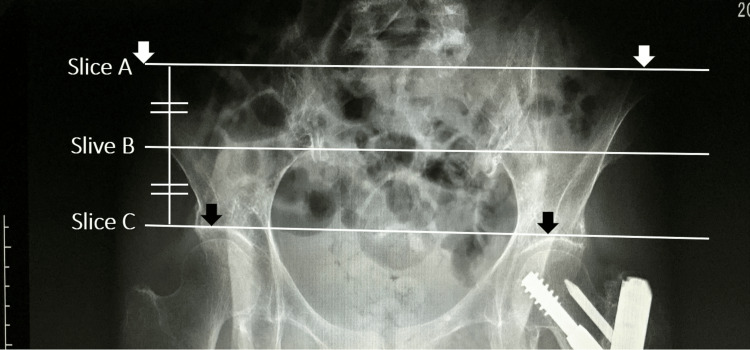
Anatomical location of the three computed tomography scan image slices. Slice A: The line connecting the anterior superior iliac spine (white arrows) bilaterally represents the proximal portion of the gluteus. Slice C: The line connecting both superior margins of the acetabulum (black arrows) indicates the distal portion. Slice B: The midway line between slices A and C.
